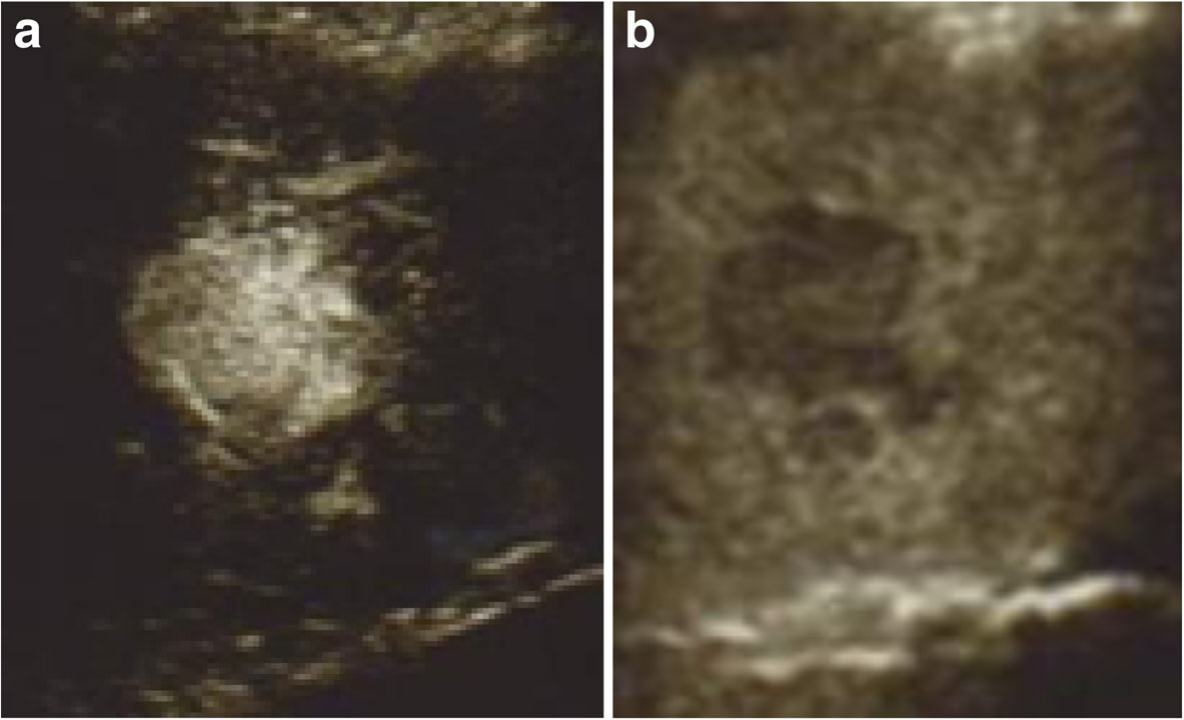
The tumor in segment VIII by enhanced ultrasound sonography at 17 weeks of gestation. a Early enhanced 32-mm tumor. b Late washed-out tumor
